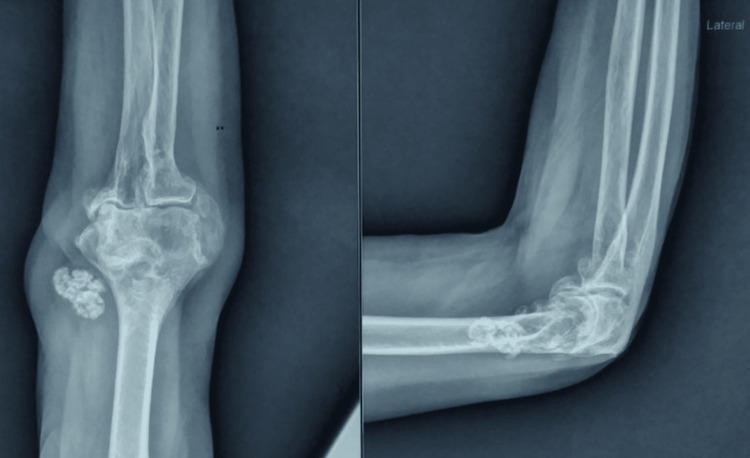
Preoperative X-ray (anteroposterior and lateral views) showing multiple loose bodies in the right elbow
